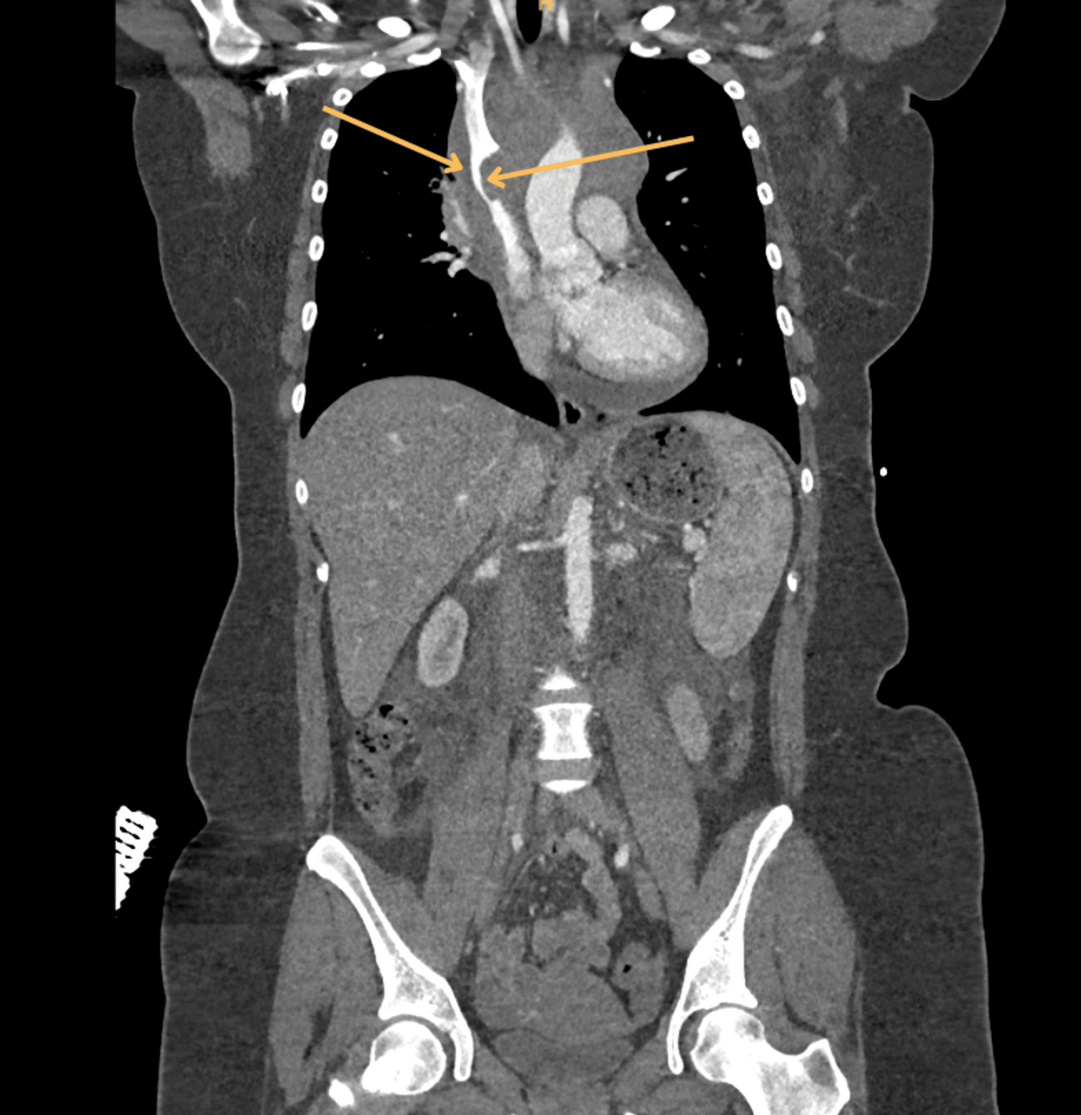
Coronal reformatted contrast-enhanced CT aortogram demonstrating a large mediastinal mass measuring 13 × 11 × 10 cm, encasing the aortic arch and causing marked extrinsic compression and narrowing of the SVC (arrows), consistent with SVC obstruction CT: computed tomography, SVC: superior vena cava
